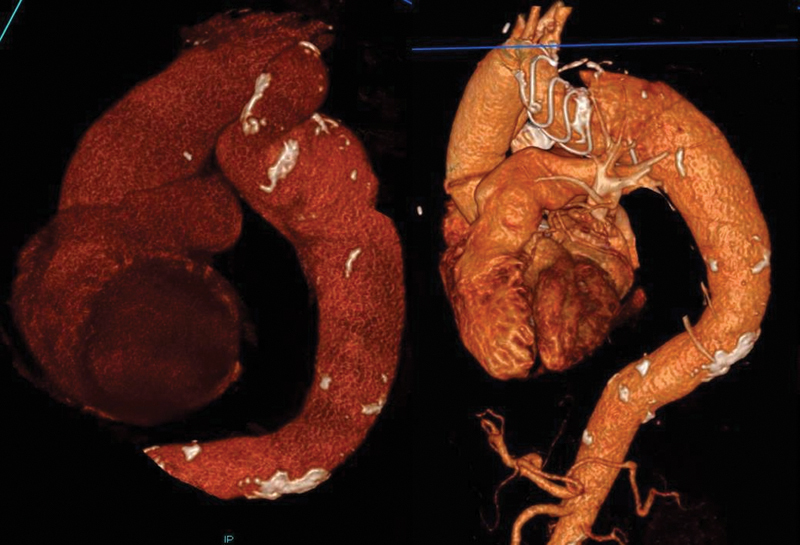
Pre- and postoperative three-dimensional rendered computed tomography imaging demonstrating severe tortuosity and the partial graft collapse. Partial graft collapse was corrected in a follow-up procedure along with the deployment of thoracic endovascular aortic repair.
